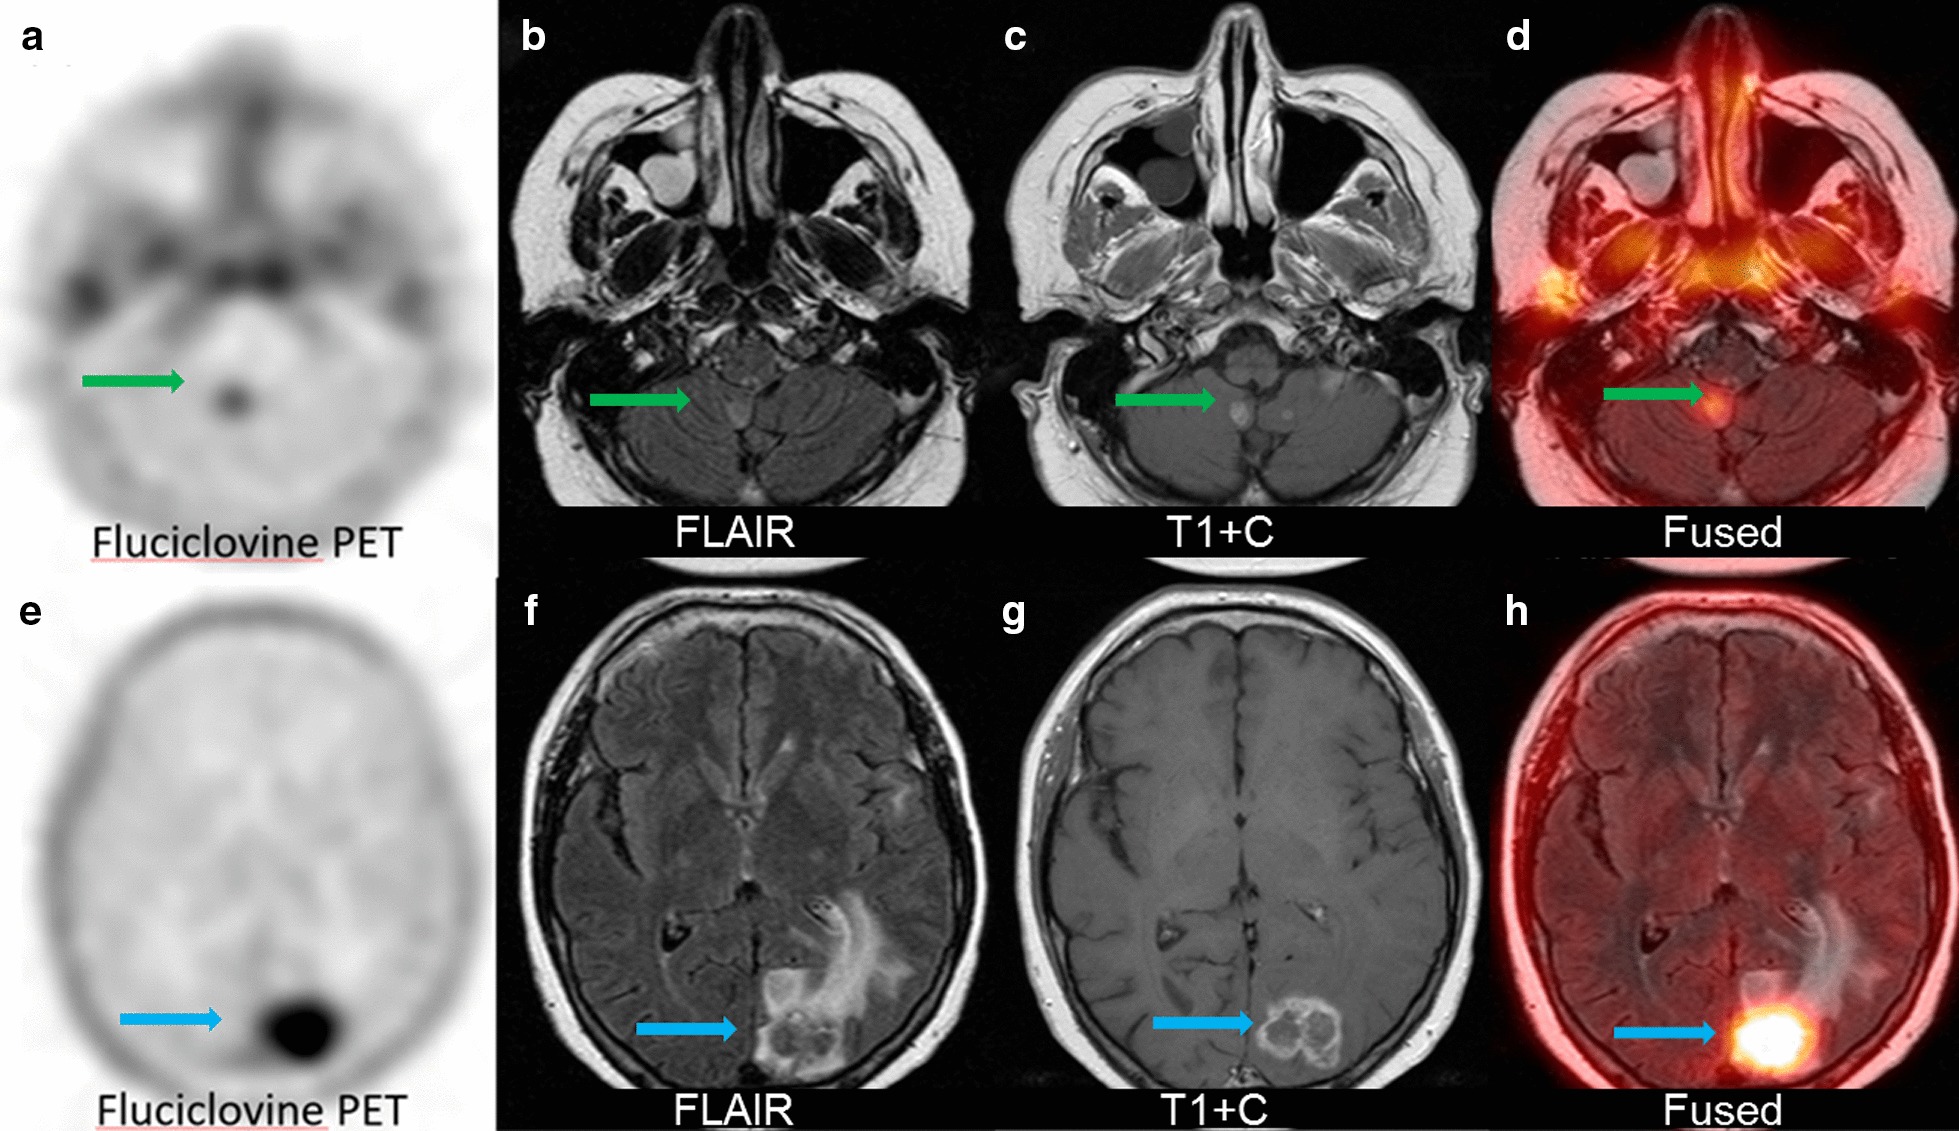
A 43-year-old patient with metastatic colon cancer with prior stereotactic radiosurgery with follow-up MRI demonstrating multiple enhancing brain lesions suspicious for recurrent disease. Top panel demonstrating a right cerebellar lesion (green arrow) with low fluciclovine uptake (SUV_max_ of 1.2) on transaxial PET (a) and corresponding focal FLAIR hyperintensity (b) T1 + contrast (c) and fused FLAIR and PET (d). This lesion did not increase in size on follow-up MRI and was consistent with radiation necrosis. A left occipital lesion (green arrow) in the same patient had high fluciclovine uptake (SUV_max_ of 2.5) on transaxial PET (e), hyperintense FLAIR (f), T1 + contrast enhancement (g), and fused FLAIR and PET (h). The left occipital lesion was found to be recurrent metastatic disease upon resection
